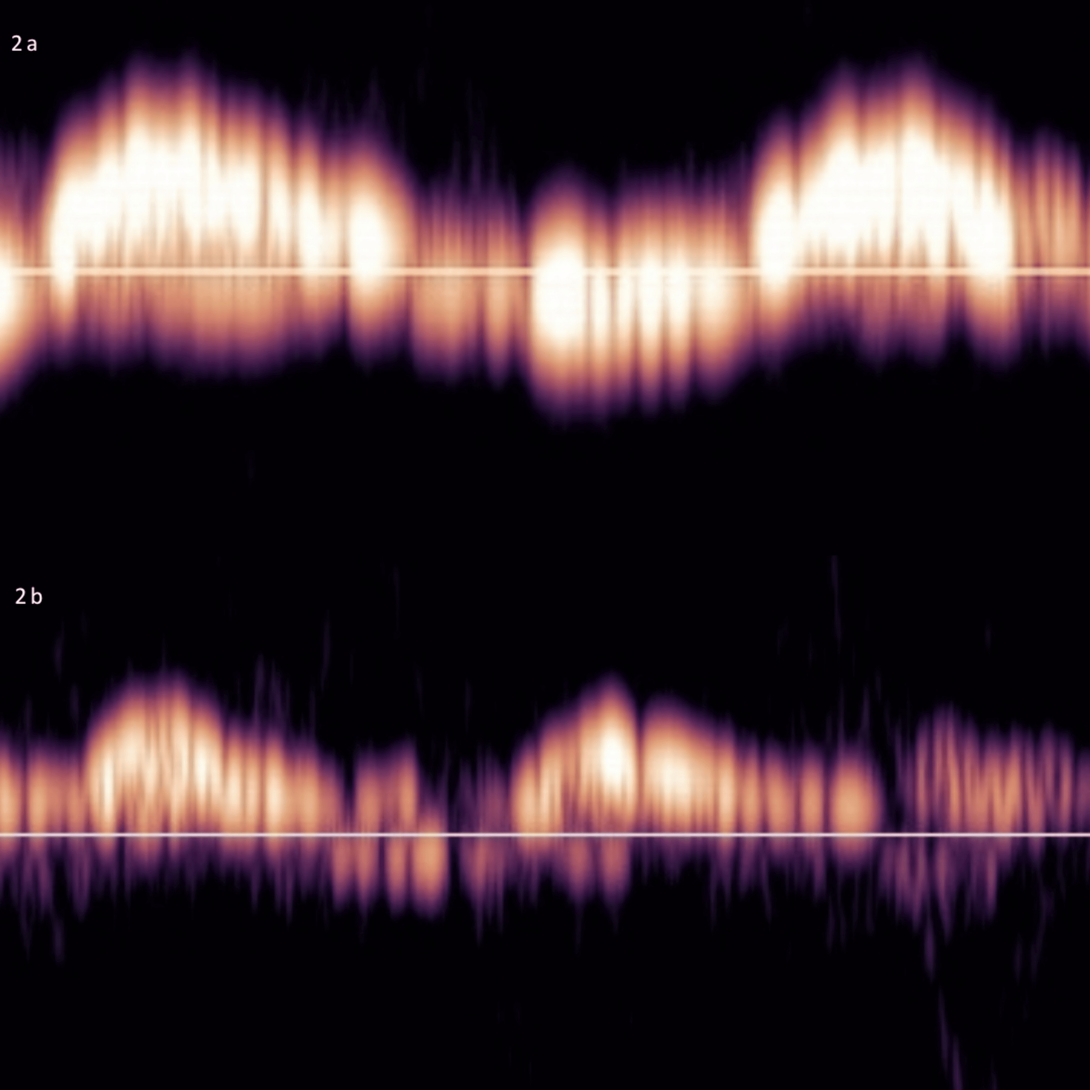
Portal vein Doppler 2a: Initial portal vein Doppler showing a >100% pulsatile Doppler waveform with flow below the baseline noted. 2b: Repeat portal vein Doppler waveform following initial fluid loss of 2400 mL with a pulsatility index of approximately 100% and significantly reduced flow below the baseline being noted.
